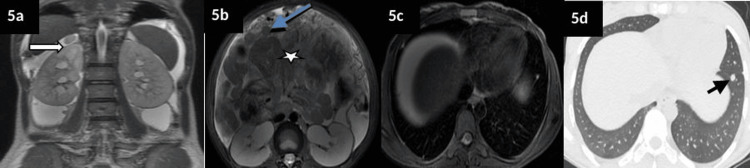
Preoperative MRI and HRCT images with metastatic deposits The coronal (a) and axial (b) T2 sequences showed moderate hydroureteronephrosis seen due to mass effect on either side with moderate ascites with small peritoneal deposits (blue arrow), omental caking (star) and right adrenal nodule (white arrow) along with small lung nodules (black arrow) in visualised lung bases on either side (c and d) of the HRCT lung window. HRCT: High-resolution CT
